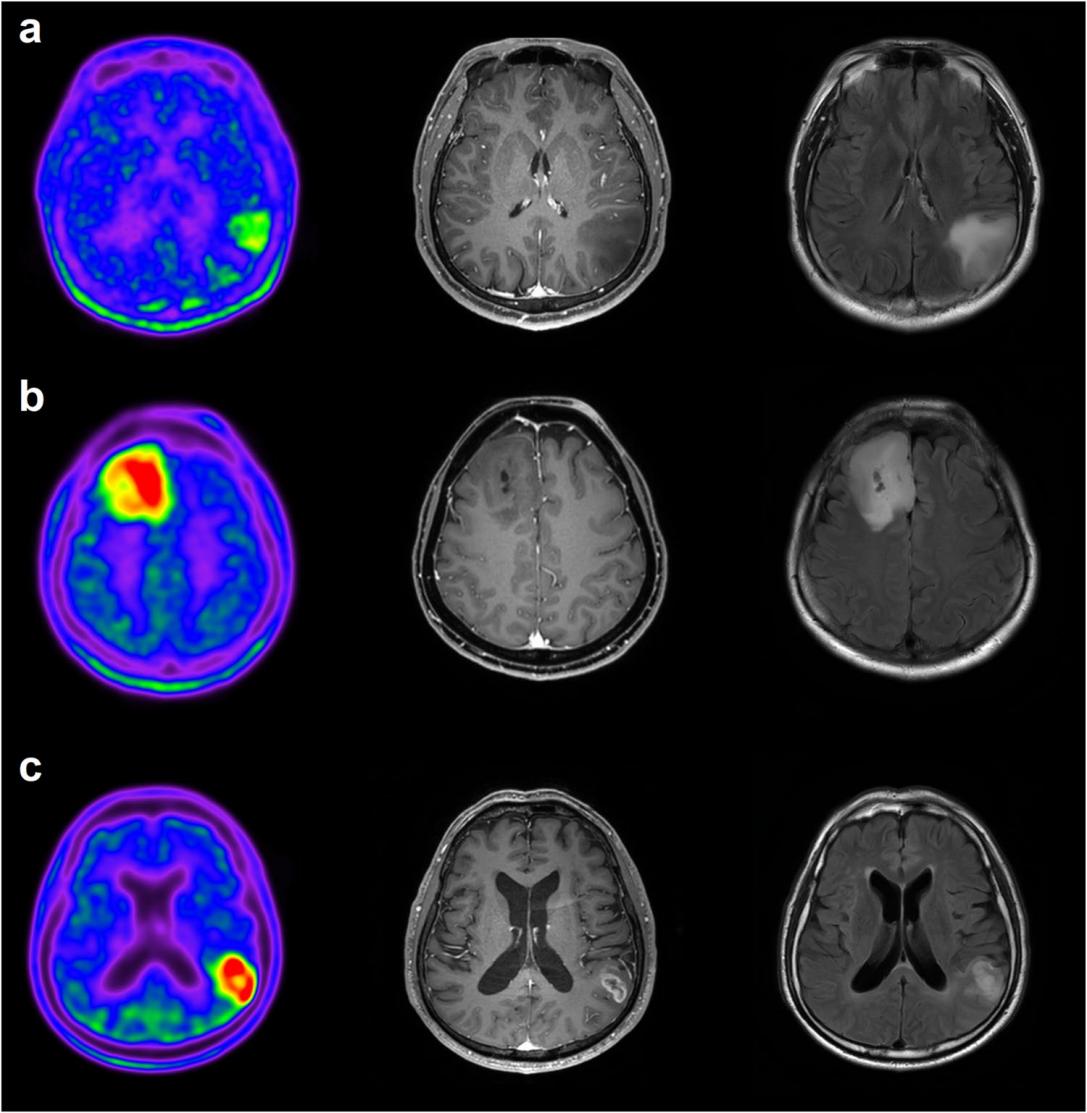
Transaxial ^11^C-methionine PET, contrast-enhanced T1-weighted MRI, and contrast-enhanced T2 FLAIR MRI images. a A patient with grade 2 astrocytoma, IDH-mutant. b A patient with a grade 3 oligodendroglioma. c A patient with glioblastoma. ^11^C-methionine has good sensitivity for detecting low-grade gliomas. However, its uptake in oligodendrogliomas is as high as in glioblastomas, which poses a significant limitation for glioma classification
